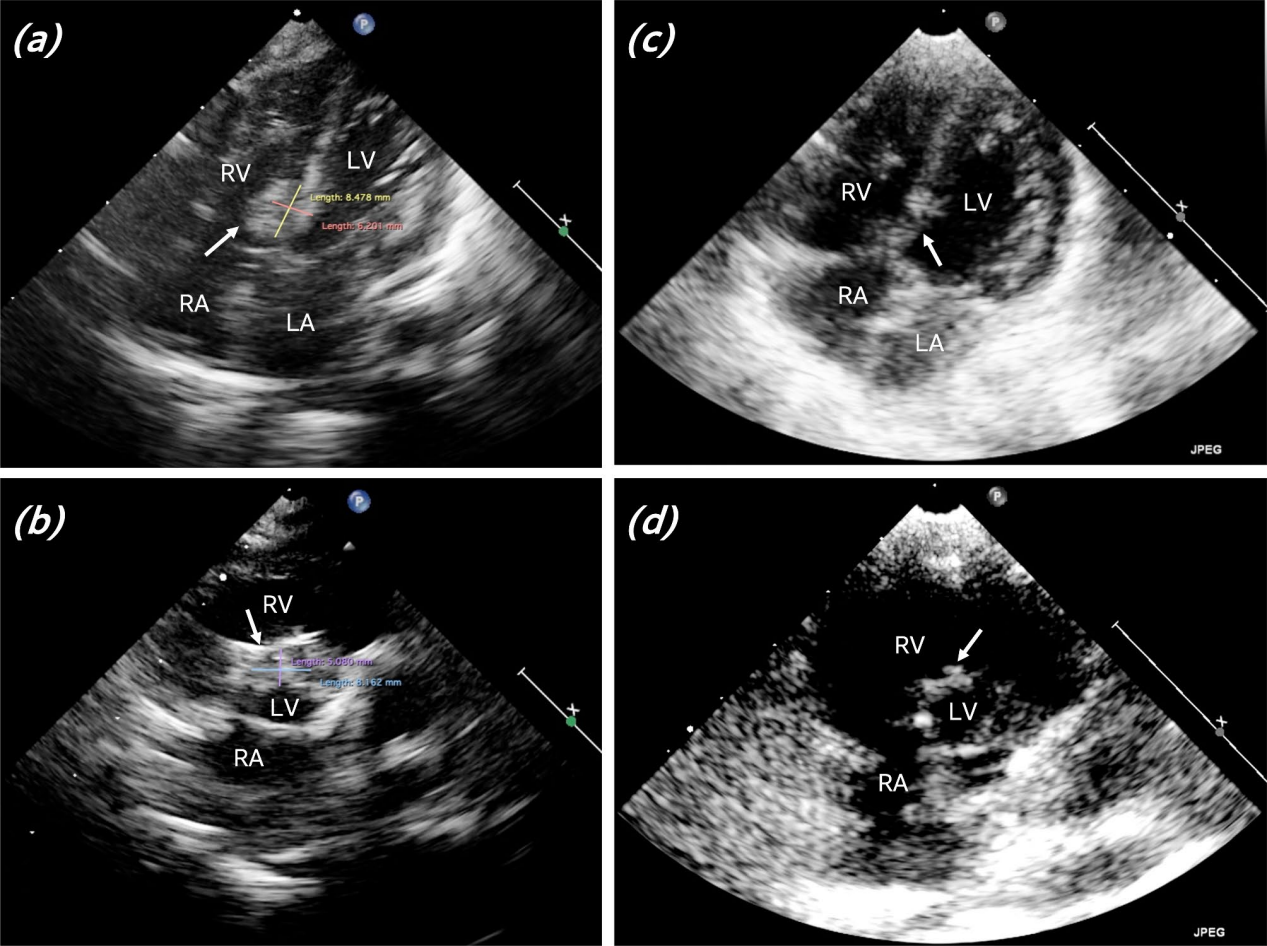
Apex five chamber (a) and parasternal short axis (b) views of TTE showing the ventricular septal defect patch (arrow) and the thrombus in the left ventricle with the maximum diameter of 8 × 6 mm. Post-treatment apex five chamber (c) and parasternal short axis (d) views of TTE showing the ventricular septal defect patch (arrow) without thrombus. LA, left atrium; RA, right atrium; LV, left ventricle; RV, right ventricle
